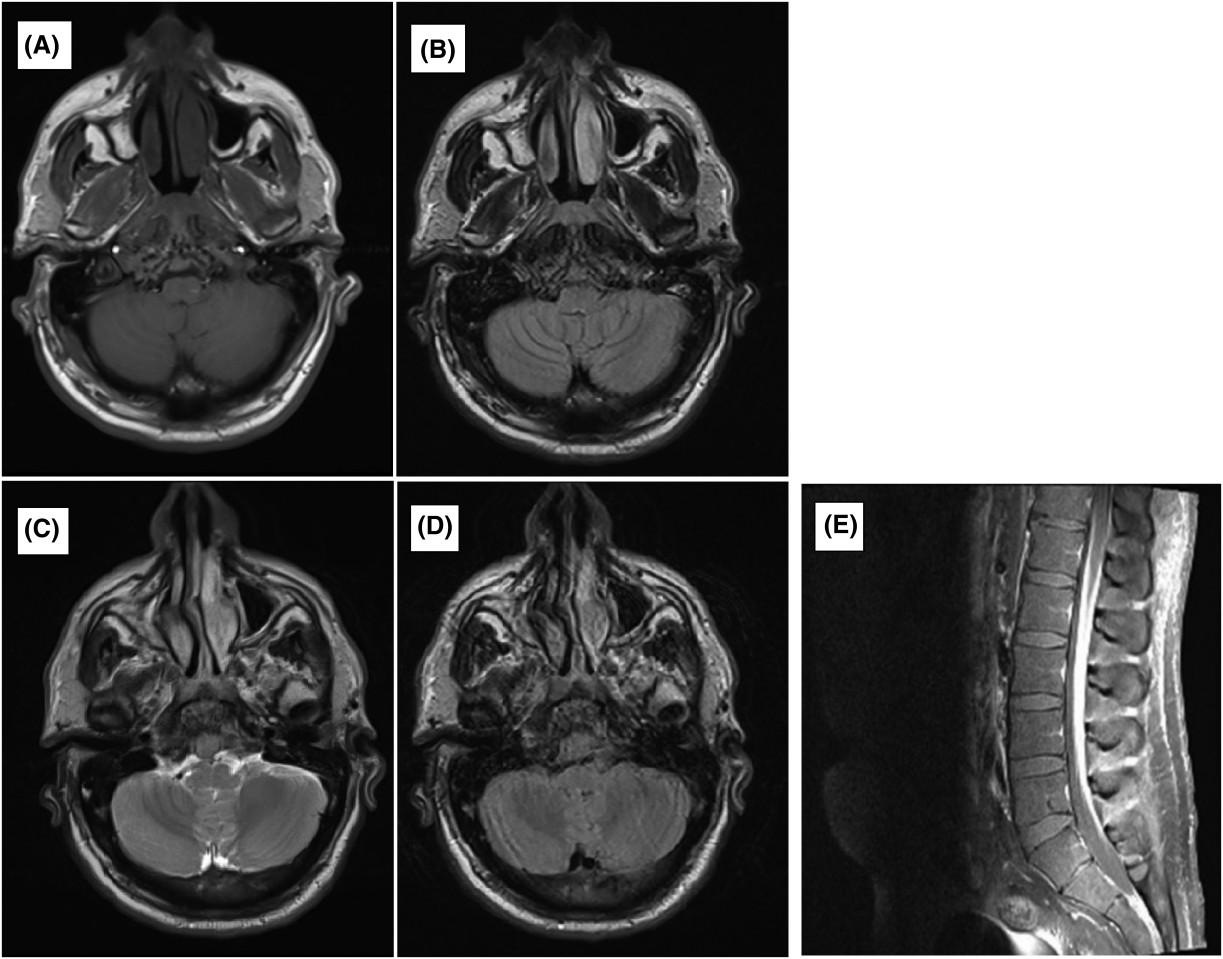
Magnetic resonance image (MRI) brain clivus mass lesion with soft mass in the prepontine cistern extending to the sphenoid and occipital condyle, involving both cavernous sinus tentorium of cerebelli and invading the pituitary gland (A: T1 pre‐contrast; B: FLAIR; C: T2, D: Post‐contrast). (E) Sagittal selected image of the lumbosacral spine (T1 post contrast) with appreciable increased enhancement along the cauda equina roots and surrounding the conus medullaris.
